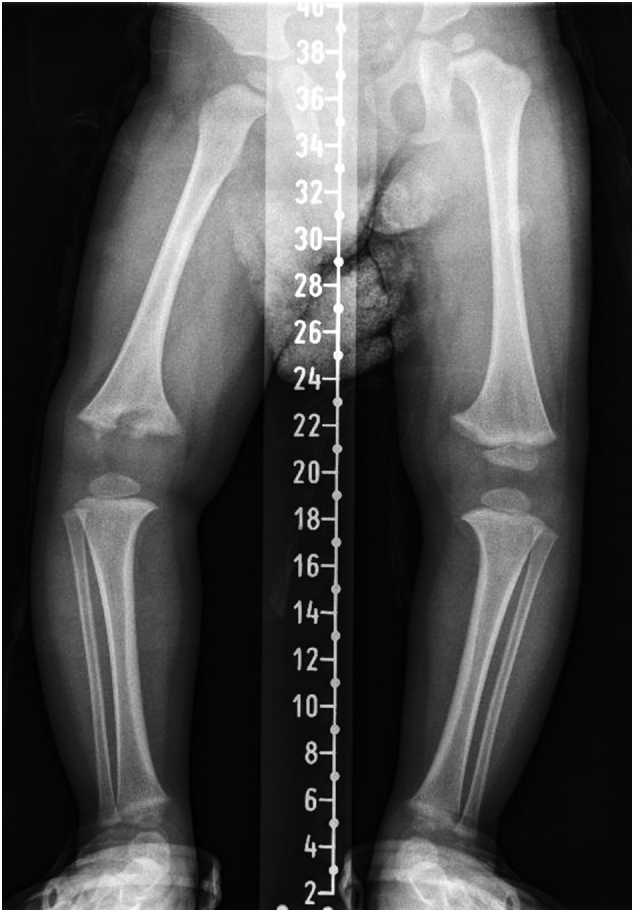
A 21-month-old boy radiograph long leg stitch view X-ray. The X-ray showed a destruction at the right distal femur growth plate.
